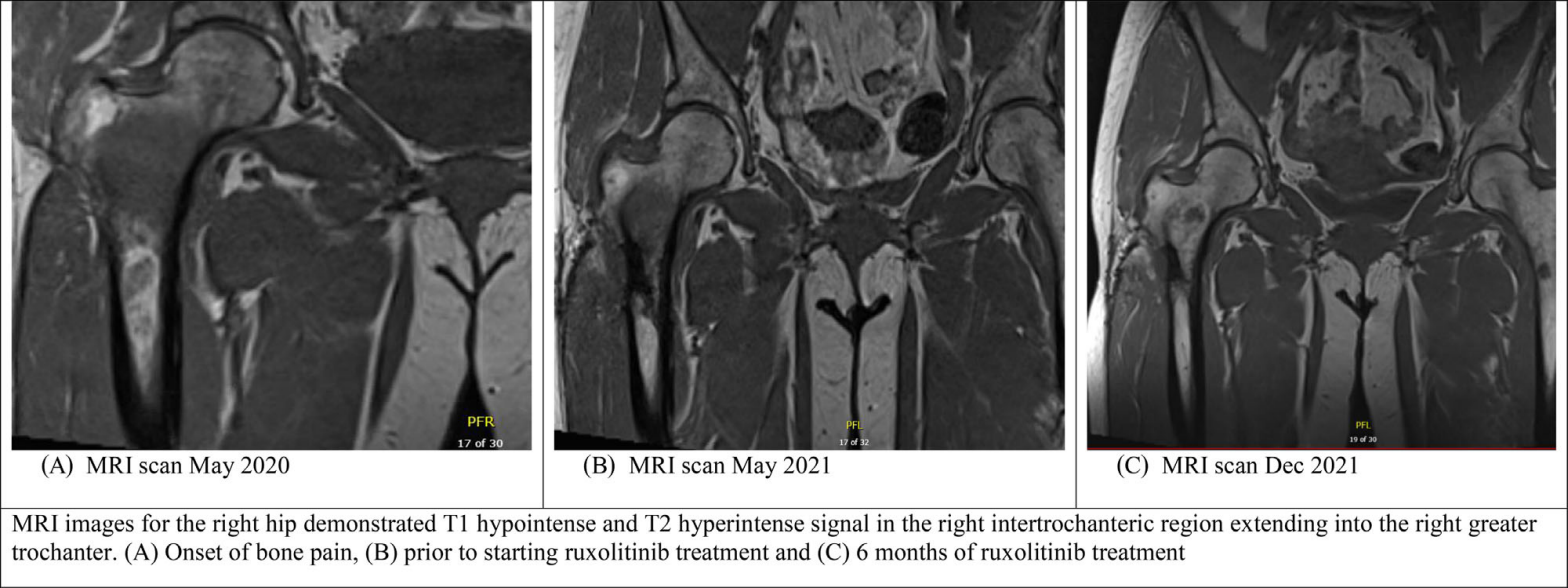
Magnetic resonance imaging (MRI) scans of the right femur
